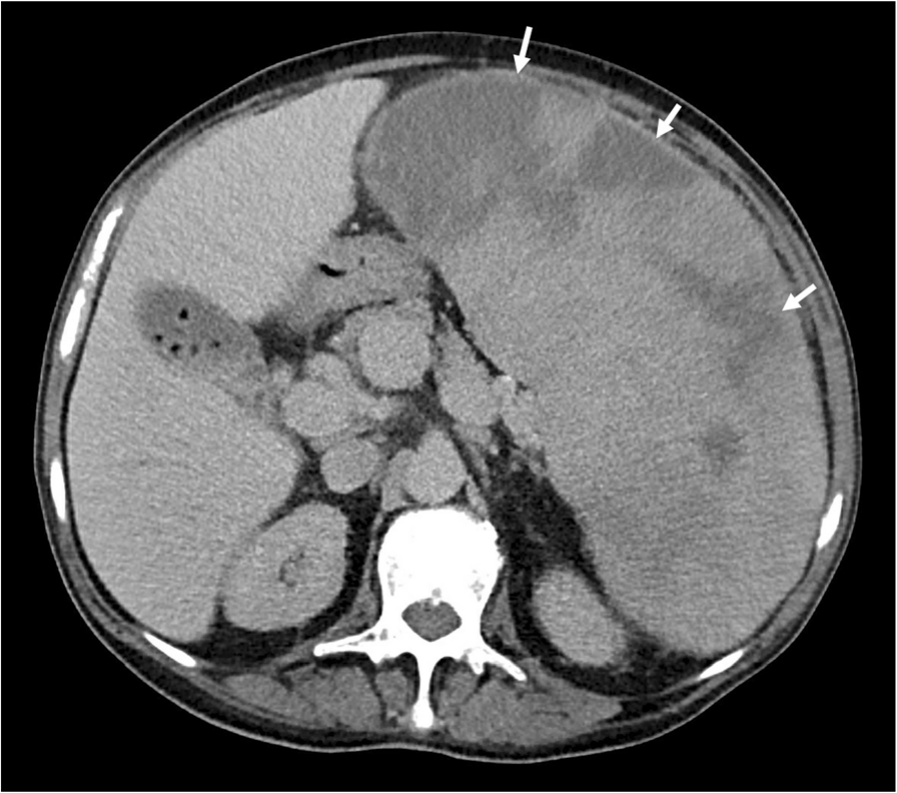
Axial portal venous phase CT of the abdomen demonstrates a markedly enlarged spleen with multiple wedge-shaped and linear areas of low attenuation consistent with splenic infarctions (white arrows). Marked enlargement of the spleen and liver may result in infarction, portal hypertension, hypersplenism, plasma volume expansion, and splanchnic vein thrombosis
